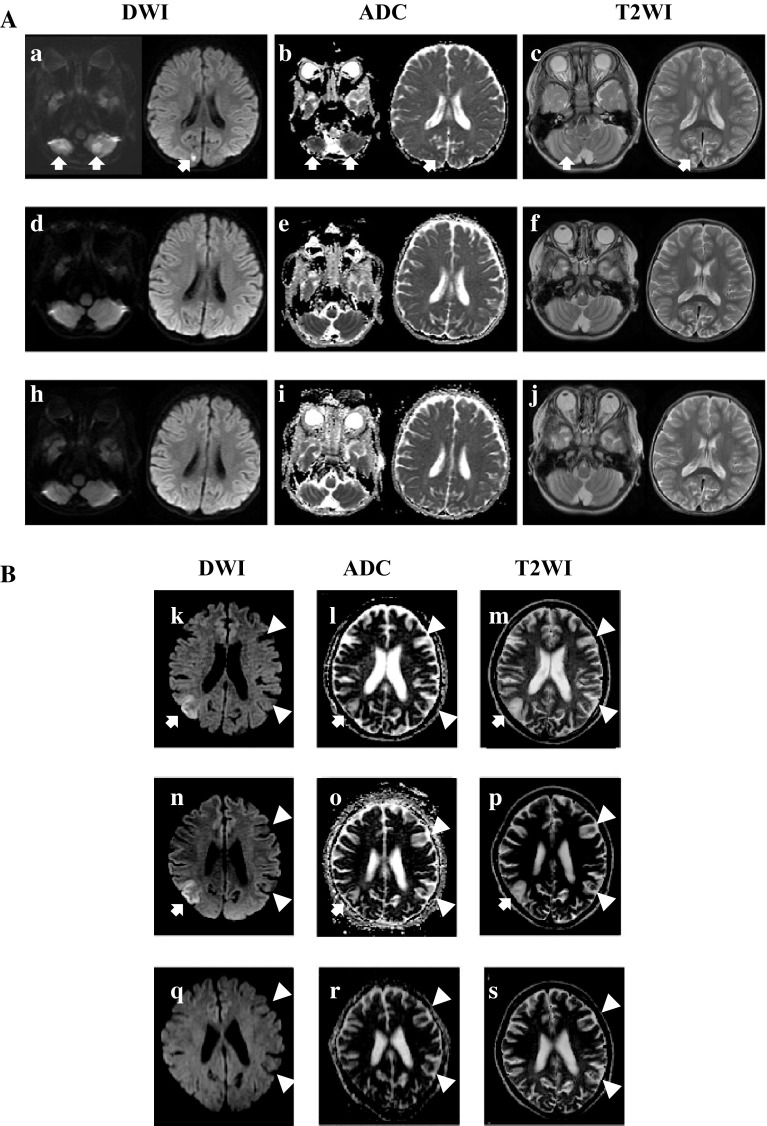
Serial brain MRI images in two MELAS patients. A A serial brain MRI was taken at 18 h (*a*–*c*), 7 days (*d*–*f*), and at 1 month (*h*–*J*) after the onset of SLE in patient 1. DWI showed high intensity signal in bilateral cerebellar cortex and right posterior cortex (*arrow*), where is mild-intensity signal in T2WI, and low intensity in ADC. However those showing abnormal intensity in 2 h after onset of SLE showed normal intensity in DWI, ADC and T2WI at 7 days and at 1 month after the episode. B A serial brain MRI was taken at 3 h (*k*–*m*), 3 days (*n*–*p*), and at 1 month (*q*–*s*) after the onset of SLE in patient 2. T2WI showed mild high-intensity signal in cortex and subcortical white matter in the right parietal lobe (*m*), which become much less high-intensity signal in 3 days (*p*), and become completely normal in 1 month after the onset of SLE (*s*). DWI showed string-like gyriform-hyperintensity localized in the cerebral cortex (*k*, *n*), which harmonized with low intensity signal on ADC map (*l*, *o*). These data suggested that cytotoxic edema exist in the cortex, on the other hand, vasogenic edema exist in the adjacent white matter. However such MRI abnormalities are not recognized in MRI images at 1 month later from the onset of SLE without atrophic change. ADC values of cortex and subcortical white matter in affected gyrus are 0.73 and 1.53 in 3 h, 0.64 and 1.51 in 3 days, and 0.86, and 0.92 in 1 month after the onset of SLE. ADC values are expressed as 10^−3^ mm^2^/s. High intensity signal in cortex and subcortical white matter in the left frontal and left temporal lobe (*white arrowhead*) (ADC and T2WI) seen in patient 2 was the old region of SLE 2 years ago, which was low intensity signal in DWI. *a*, *d*, *h*, *k*, *n*, and *q* were DWI. *b*, *e*, *i*, *l*, *o* and *r* were ADC. *c*, *f*, *j*, *m*, *p* and *s* were T2WI map
